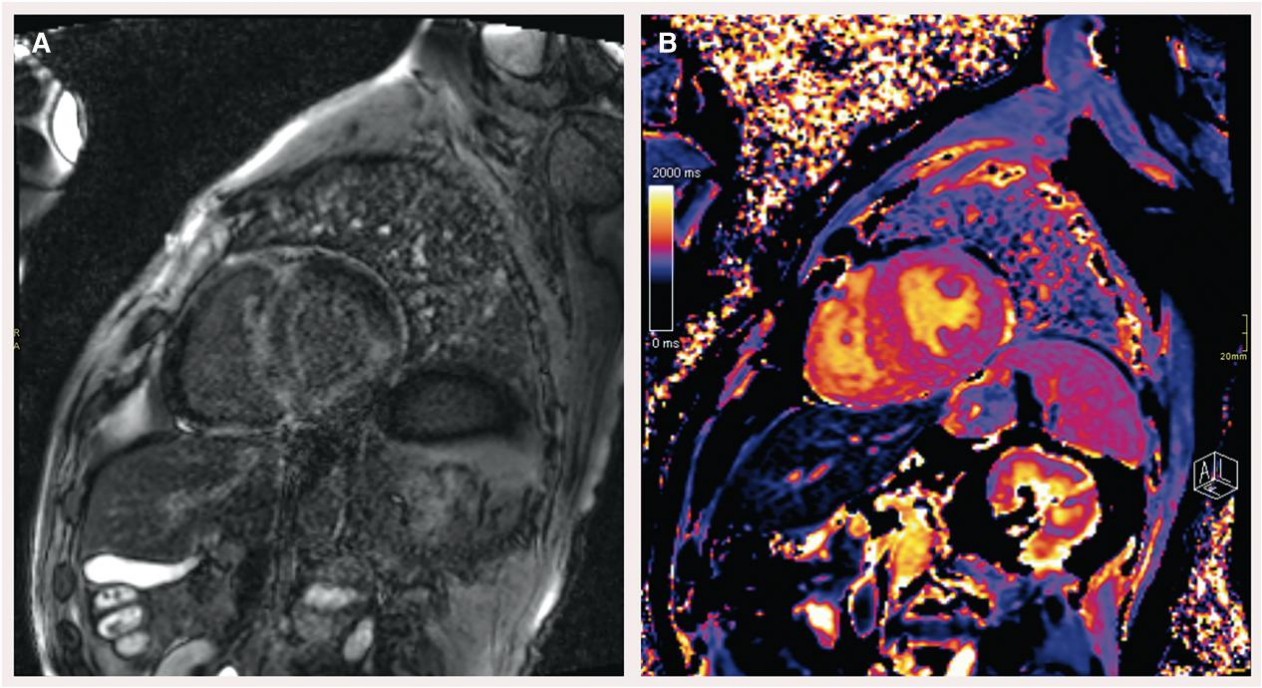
Cardiac magnetic resonance imaging. *(A)* Diffuse late gadolinium enhancement (LGE), pathological contrast uptake in the left ventricular myocardium. Mainly seen subendocardial and subepicardial. Suspected similar contrast uptake in the right ventricular wall. *(B)* Diffusely increased T1 relaxation time in the left ventricular wall, primarily visible in the septal and inferolateral regions at the basal area.
